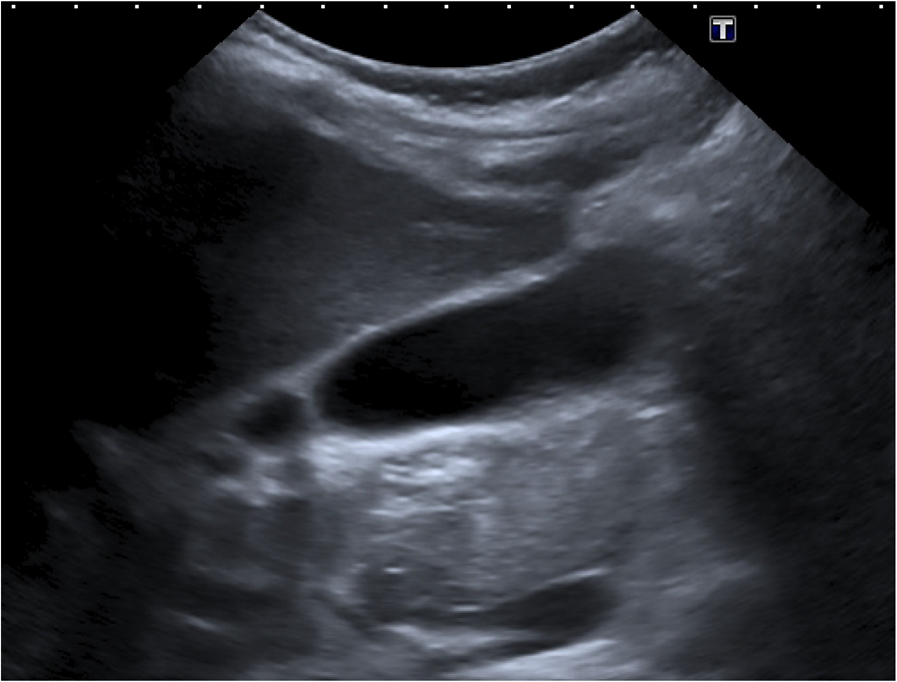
Transabdominal Ultrasound of the Gallbladder after regression of clinical symptoms. Toshiba Aplio 500
